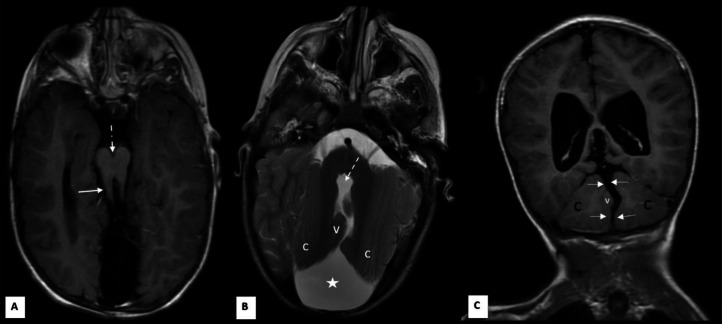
Joubert Plus syndrome. Axial T1-weighted (A), axial T2-weighted (B), and coronal T1-weighted (C) MRI images. Panel (A) demonstrates the elongated superior cerebellar peduncles (solid arrow) and deep interpeduncular fossa (dashed arrow), characteristic of the molar tooth sign. Panels (B and C) show the fourth ventricle in direct communication with a large CSF-filled posterior fossa cyst (five-point star), resulting in thinning of the overlying occipital calvarium. In panel (C) the cerebellar hemispheres (highlighted with the letter “c”) are separated by a deep midline cleft and exhibit an interdigitating appearance (solid arrows). Notice the vermis appears markedly hypogenetic with elevated fastigium, denoted by the letter “V”.
